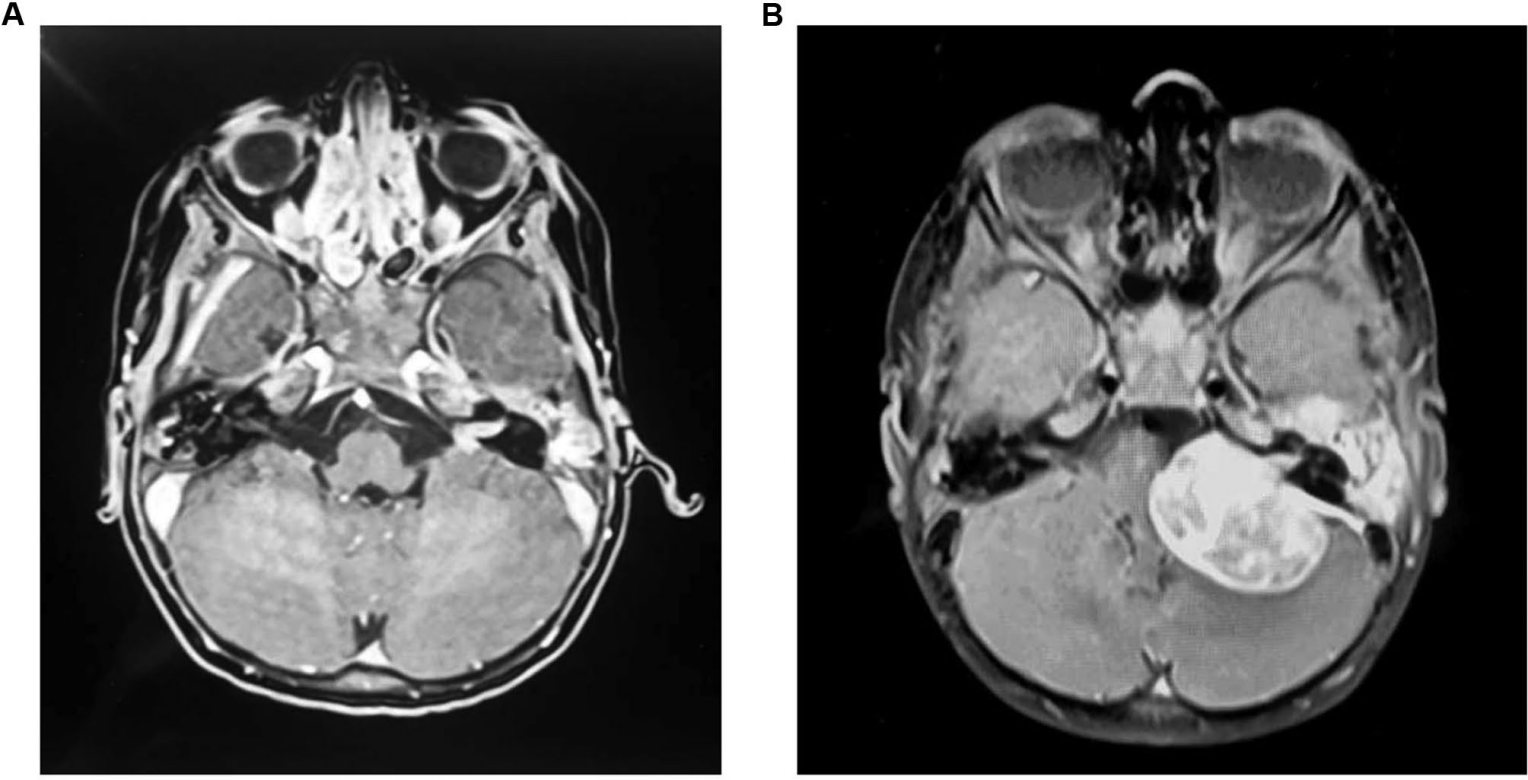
a Initial T1 post contrast MRI demonstrating 7 mm × 11 mm enhancing lesion in the left internal acoustic meatus b Follow-up T1 post contrast MRI demonstrating 4.0 cm × 4.0 cm × 3.3 cm on the left side and an enhancing lesion in the right temporal bone
